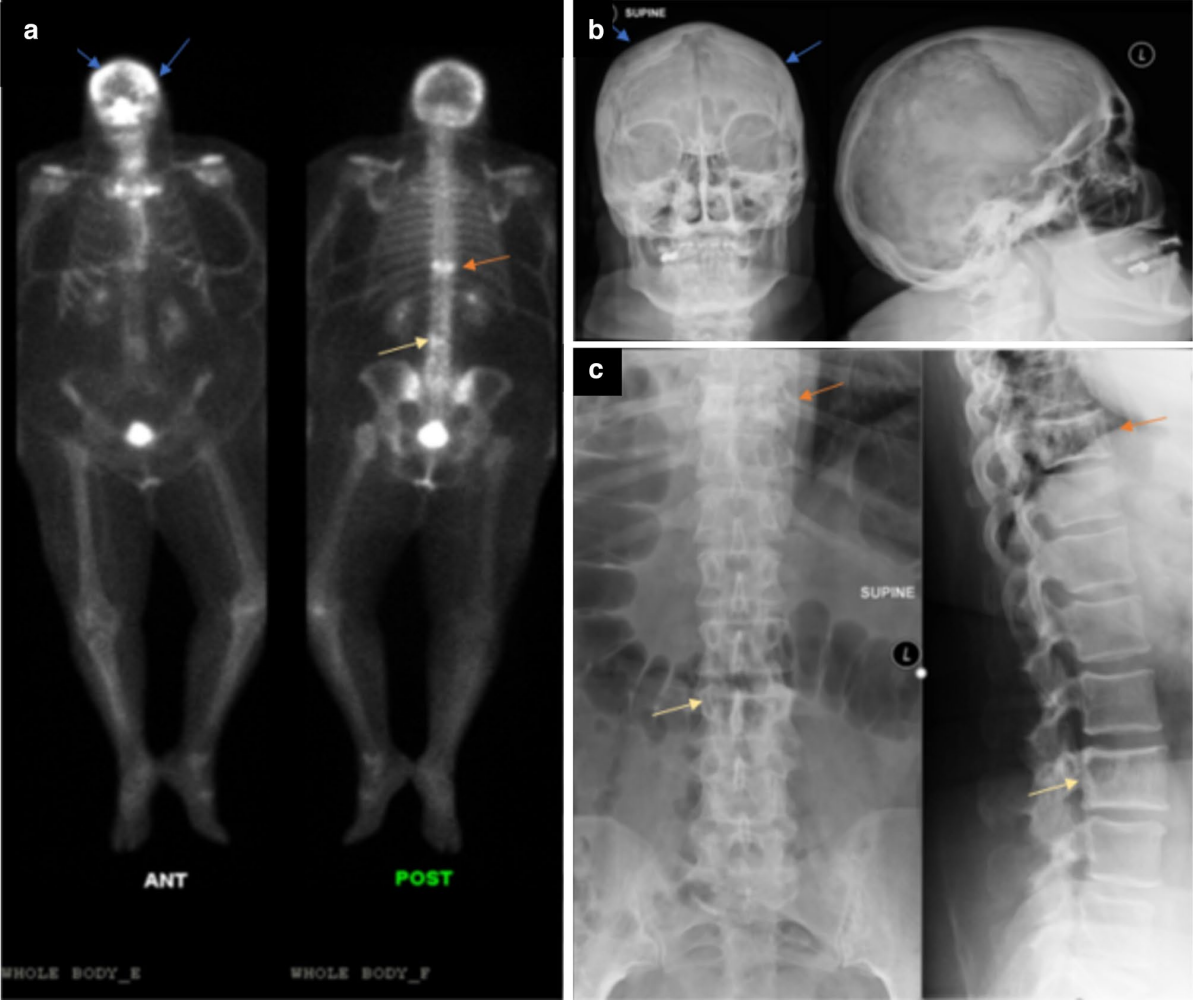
Whole body 99mTc-MDP bone Scan and Radiographic findings in patient 1 (ID 1). A 60-years-old female with back pain and leg pain, limb girdle distribution of myopathy and Paget disease revealed (a) increased uptake in the skull, thoracic and lumbar vertebrae on whole-body bone scan, increased uptake in the knees due to associated arthritis, (b) radiograph images reveal corresponding bone lesions of the skull bilaterally (blue arrows), (c) thoracic T10 vertebrae (orange arrow) and lumbar L3 vertebrae (yellow arrow).
